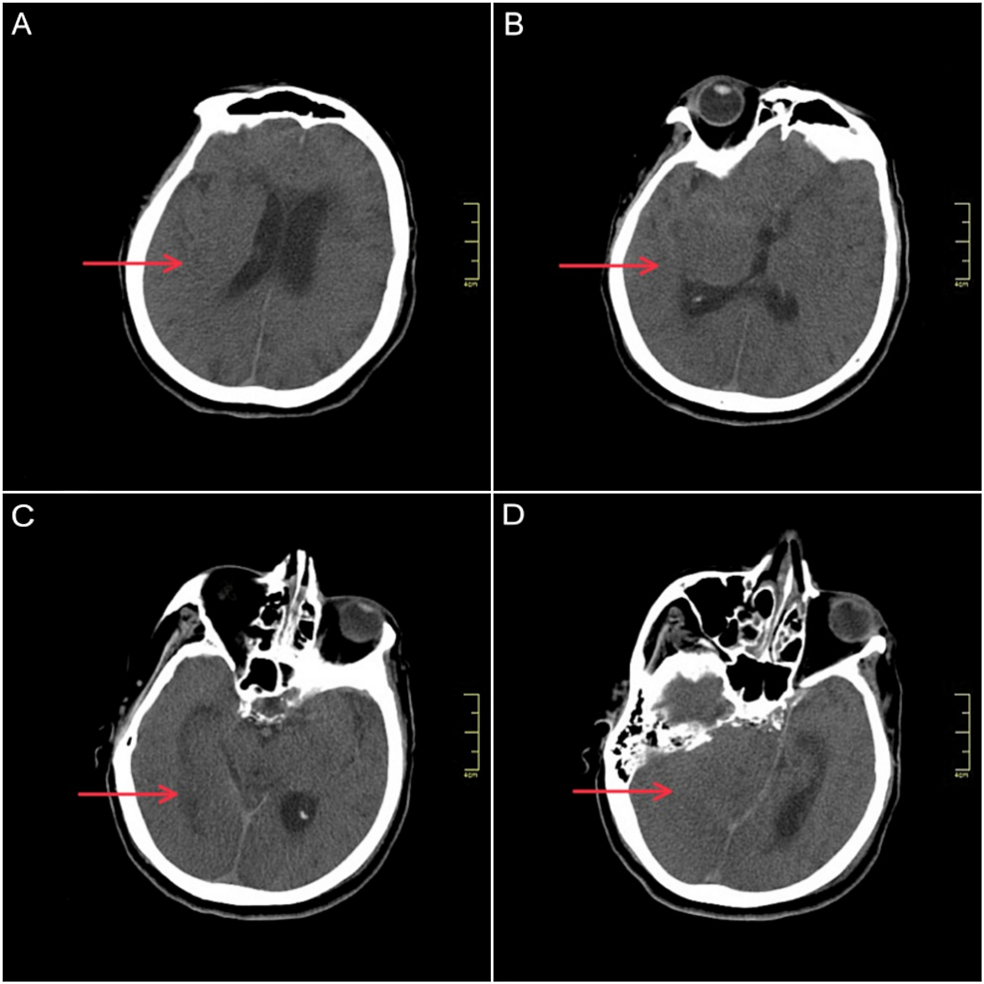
Head CT showing cerebral edema A, B, D: Red arrows show diffuse loss of grey-white differentiation and sulcal effacement; C: The red arrow shows slight dilatation of the temporal horn of the lateral ventricle
